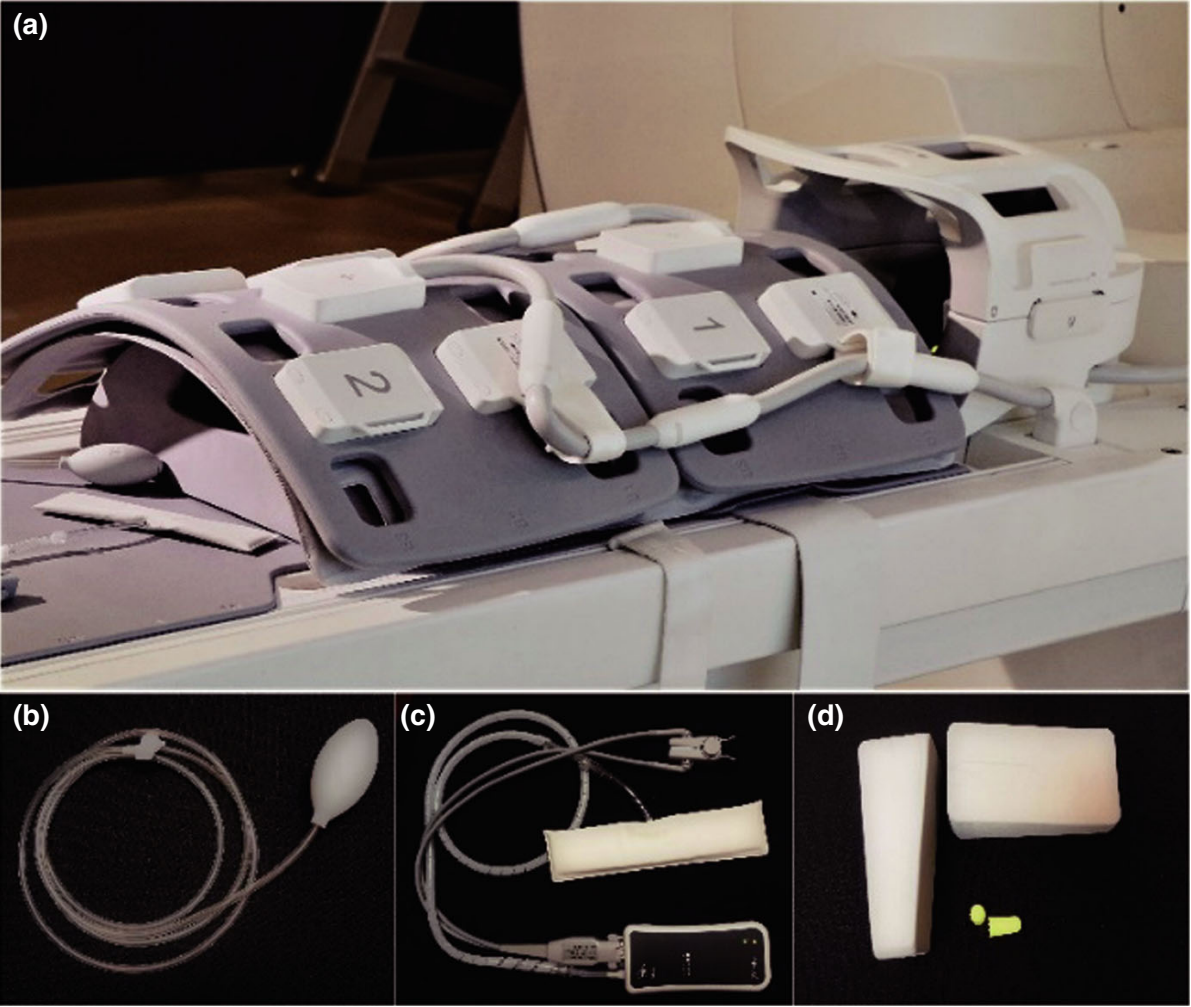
Coils and accessories, (a) dedicated PET/MRI whole‐body coil and head coil, (b) alarm ball, (c) vital signs monitor, and (d) wedge pad and earplugs.
